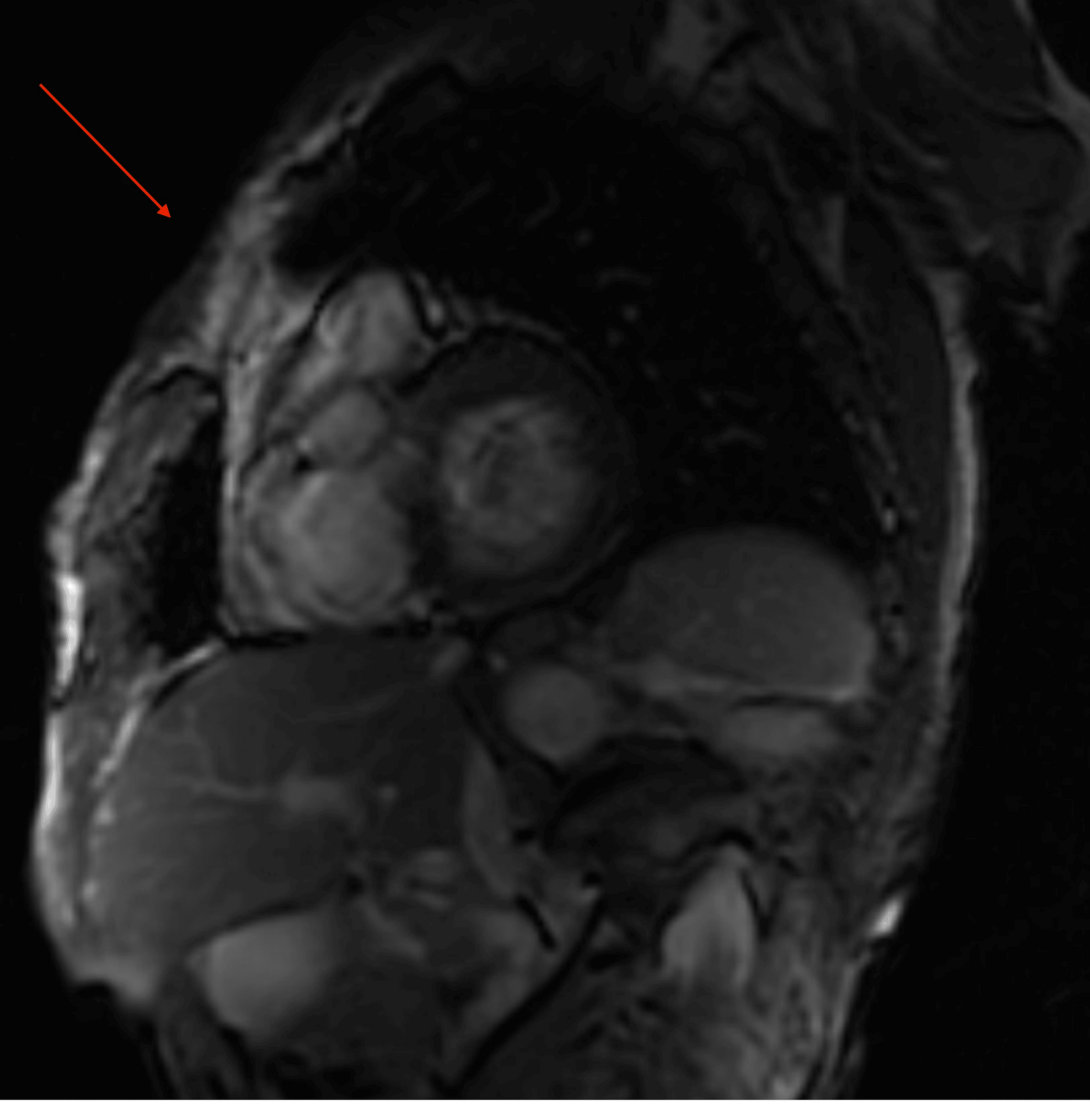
Cardiac MRI (sagittal view) showing a mass (red arrow) attached to the mitral annulus
